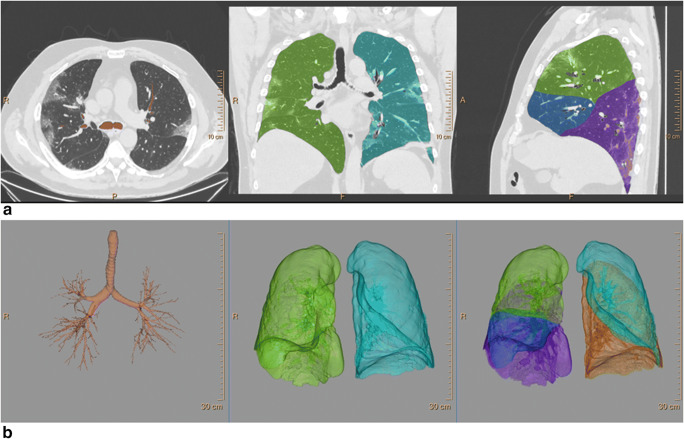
Automatic lobe segmentation process, derived from native unenhanced CT images in axial, coronal, and sagittal planes (a right, middle, and left panel, respectively), and the sequential segmentation of airways, lungs, and lobes (b right, middle, and left panel, respectively) in a 65-year-old man with SARS-CoV-2-related pneumonia
